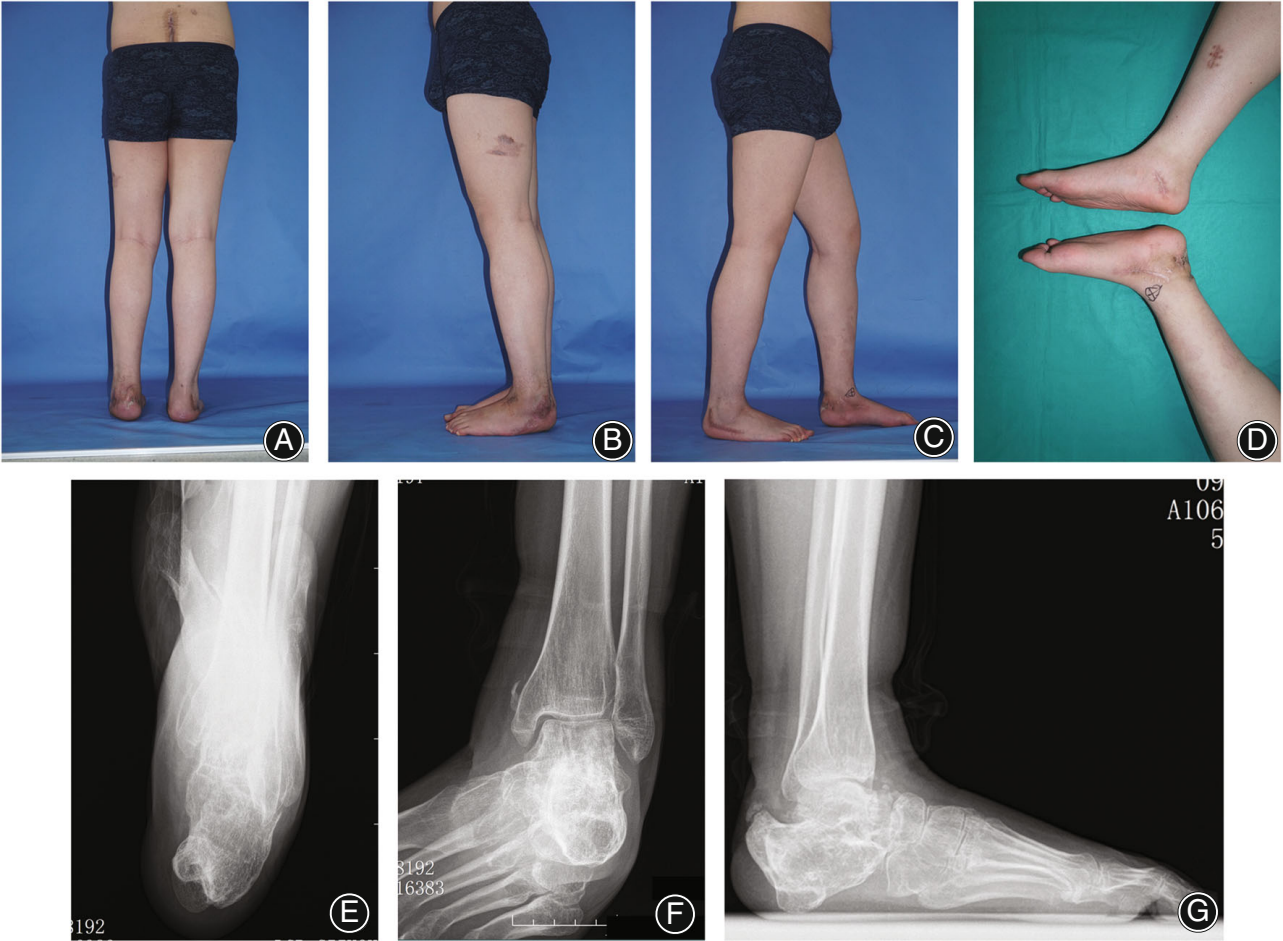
Preoperative appearance and radiography of the typical case. (A–D) The affected foot and ankle were in poor appearance with obvious malleolar varus and hindfoot deficiency. (E–G) Fluoroscopy showed a defect calcaneus with abnormal calcaneal interface angle (CIA), angle of a longitudinal arch (ALA), metatarsal declination angle (MDA), and tibio‐calcaneal angle (TCA).
